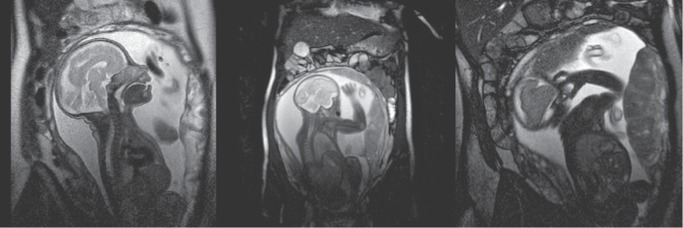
Fetal MRI (coronal plane) during pregnancy at 29 weeks' gestation. MRI, magnetic resonance imaging. Images presented with patient permission.
